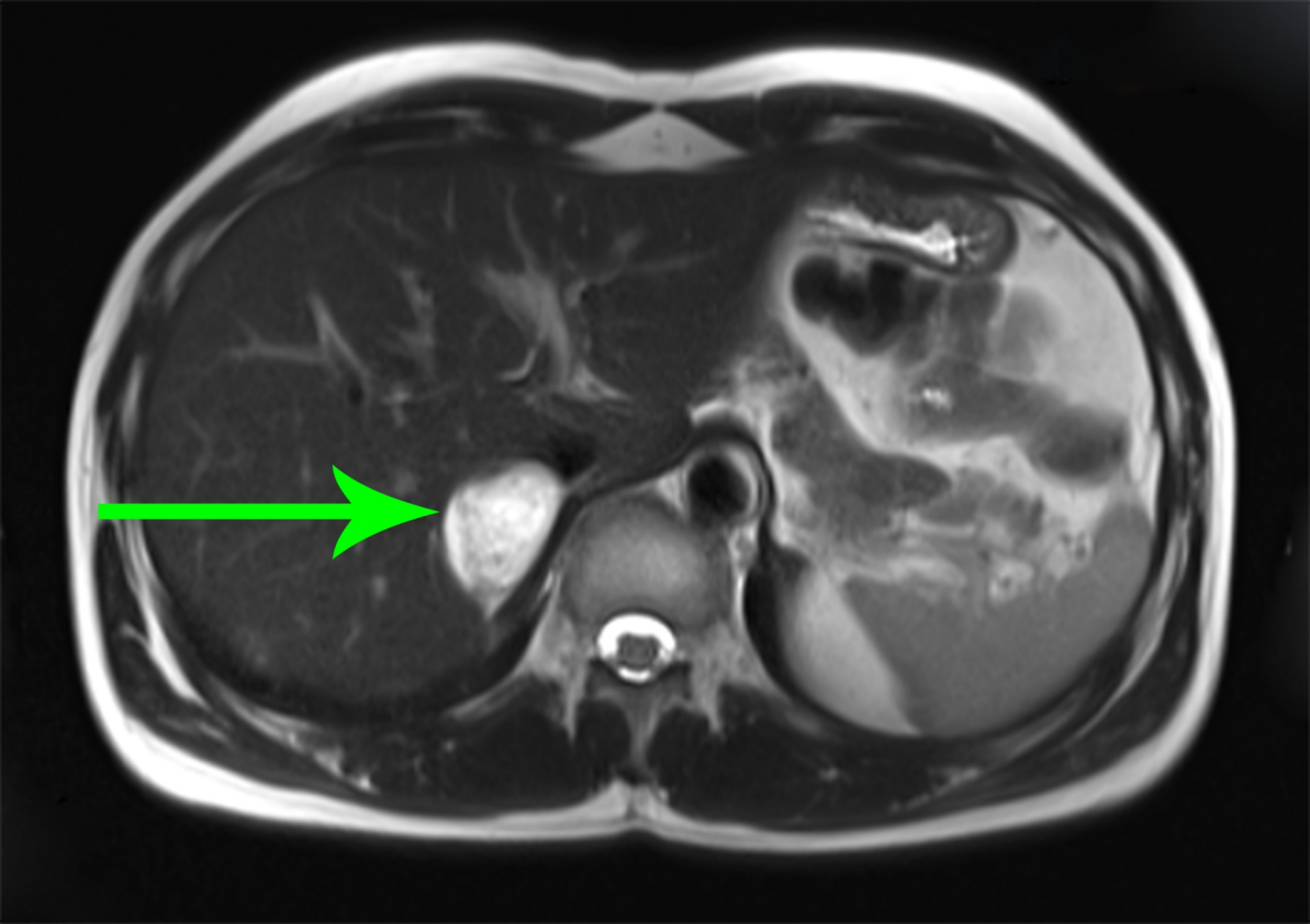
Magnetic resonance imaging (MRI) of the abdomen. The arrow points towards a right suprarenal heterogeneous mass.
